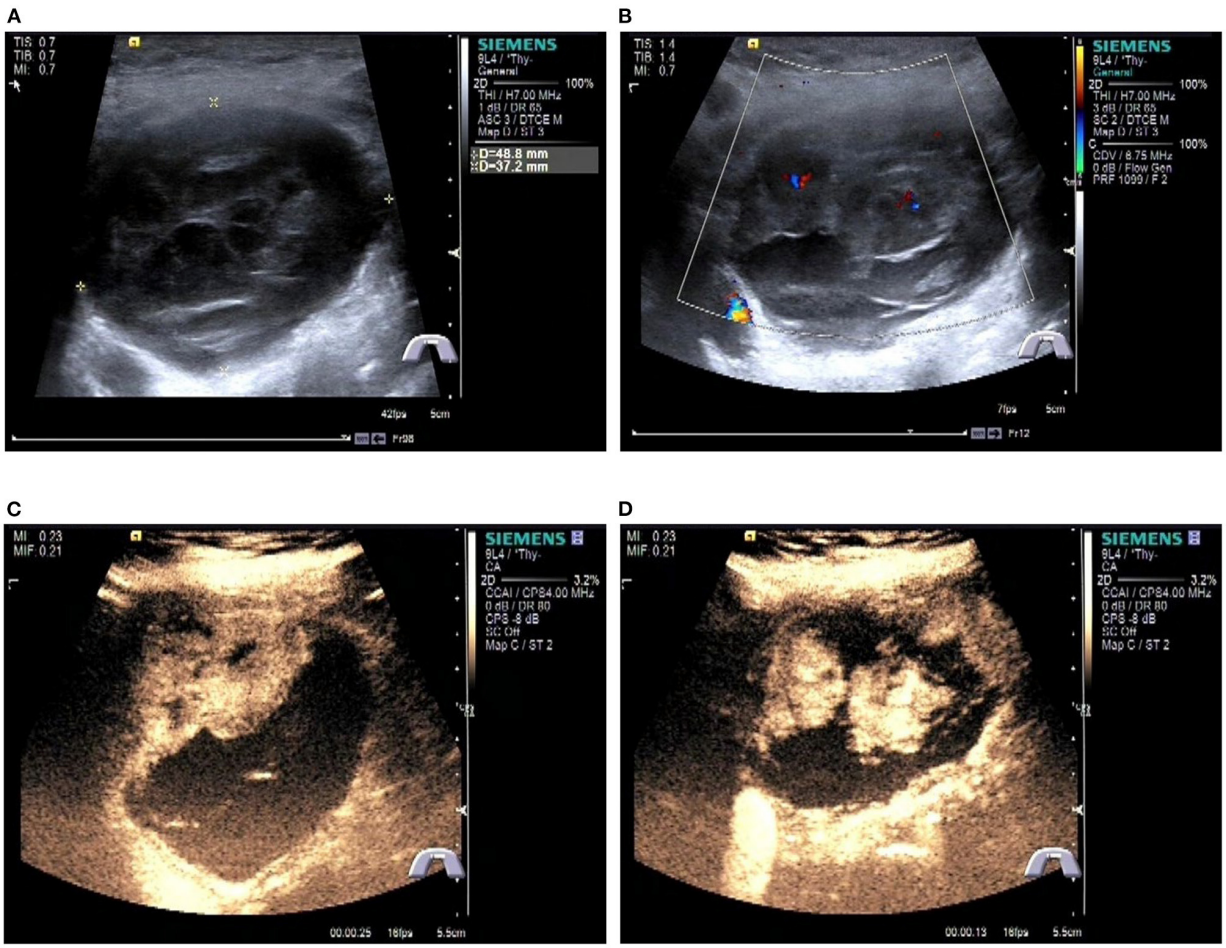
Preoperative contrast-enhanced ultrasonography of the patient. (A) Gray scale ultrasonography of the mass. (B) Color Doppler imaging of the mass. (C, D) Contrast-enhanced ultrasonography of the tumor.
